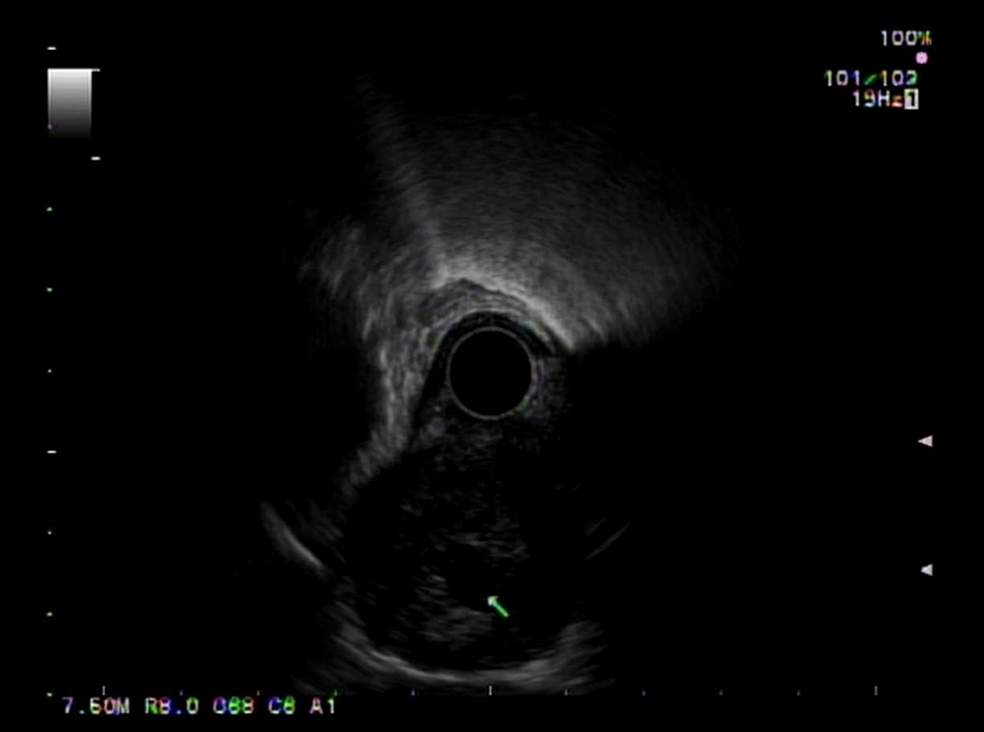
Endoscopic ultrasound view of the esophageal schwannoma hypoechoic lesion with anechoic cystic space.
